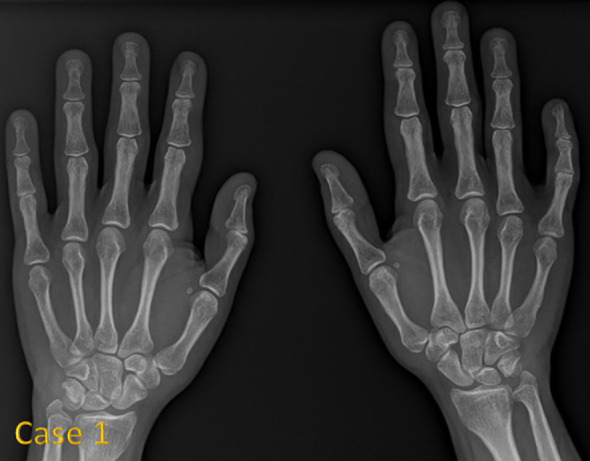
Anteroposterior radiograph of both hands in case 1 reveals slender tubular phalanges.
